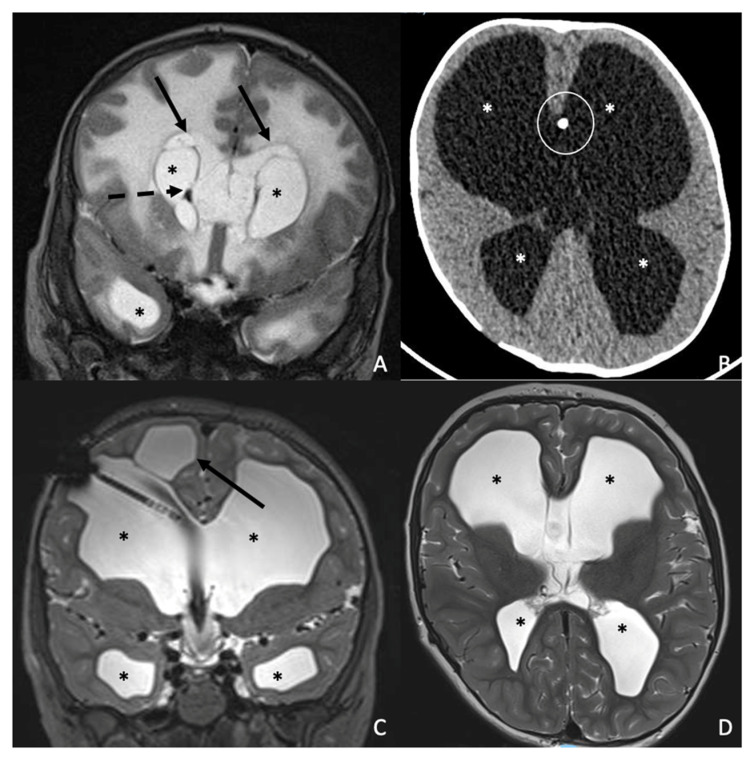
Serial neuroimaging studies in a boy diagnosed with *Listeria monocytogenes* infection. The figure includes the first MRI performed at 1 month and 10 days of age (A), followed by a CT scan at 1 month and 20 days of age (B), and the final MRI at 7 years of age (C,D). Baseline coronal T2-weighted imaging (A) shows diffuse supratentorial cortical and white-matter edema with effacement of the subarachnoid spaces at the convexities and skull base. There is marked dilatation of the lateral ventricles and third ventricle (black asterisks), with parietal ventricular-margin susceptibility consistent with hemorrhagic products/hemosiderin deposition (black dotted arrow), and multiple periventricular and deep white-matter cystic dilatations (black arrows). The non-contrast axial CT (B) shows further ventricular dilatation consistent with worsening hydrocephalus (white asterisks) and the presence of a ventriculoperitoneal shunt catheter (white circle). Follow-up MRI at 7 years of age includes coronal (C) and axial (D) T2-weighted images, showing persistent ventricular dilatation (black asterisks) with effacement of the subarachnoid spaces at the convexities and skull base, as well as the ventriculoperitoneal shunt catheter and multiple periventricular and deep white-matter cystic dilatations (black arrow).
